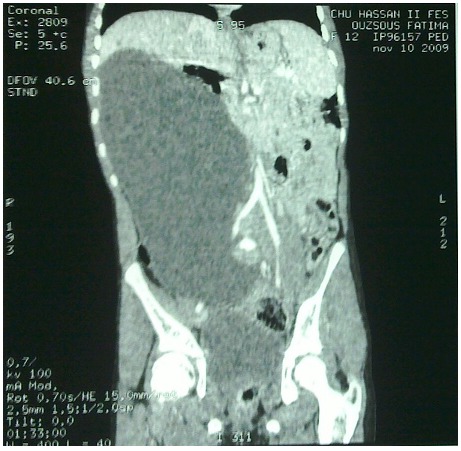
Abdominal CT scan of the same patient, coronal view
